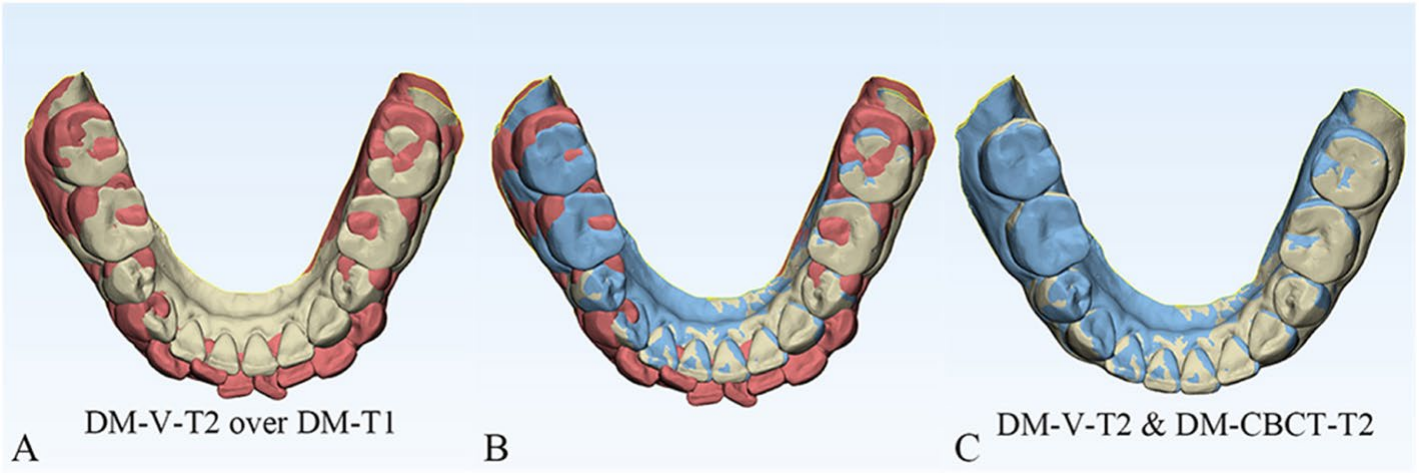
A Vessel-based DMs superimposition, registration of DM-V-T2 (yellow) to DM-T1 (red). B DM-T1 (red) and DM-CBCT-T2 (blue) and DM-V-T2 (yellow). C Top views of 2 methods of superimposition
